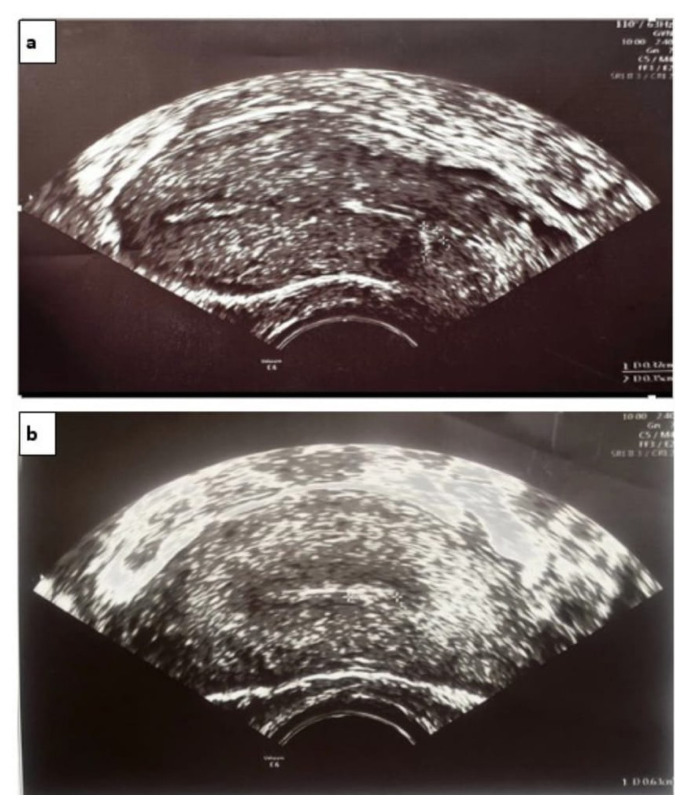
Transvaginal ultrasonographic images portraying uterine scar niche measurements at 6 months after surgery. Measurement of the width and height of the defect and appearance of the residual myometrial thickness (a); diameter of the defect (b).
